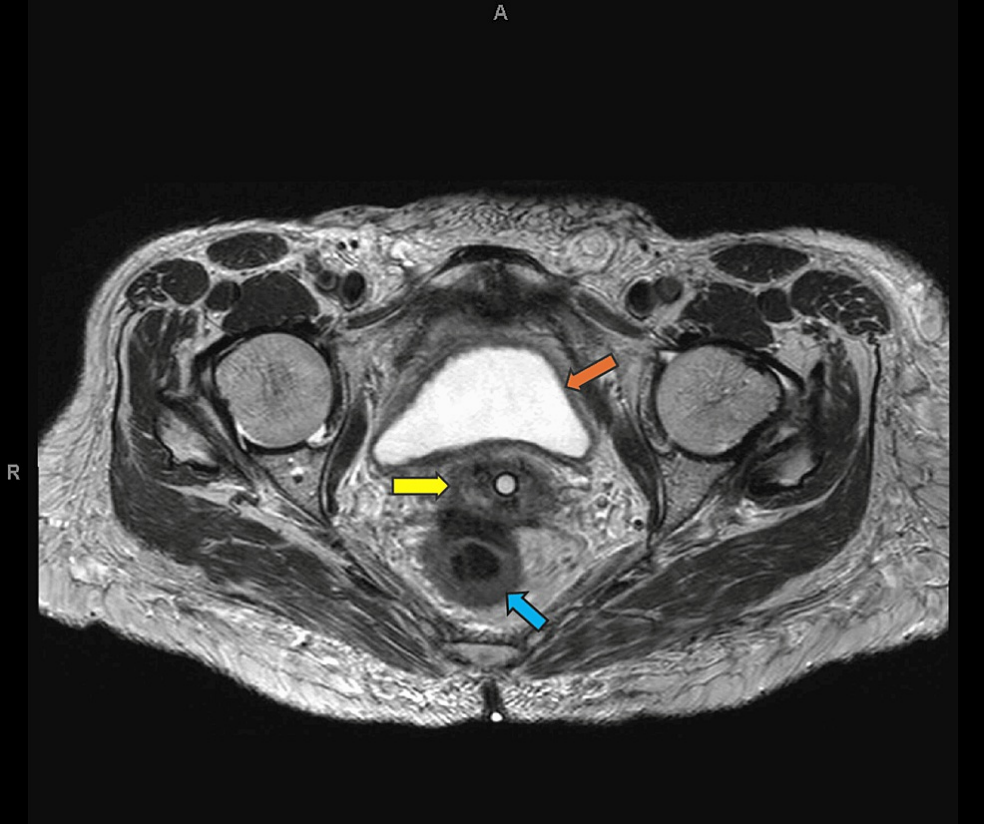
Axial MRI showing complete response to therapy with no radiographic evidence of the cervical mass Orange arrow: bladder, Yellow arrow: Cervix with no disease seen, Blue arrow: Rectum
